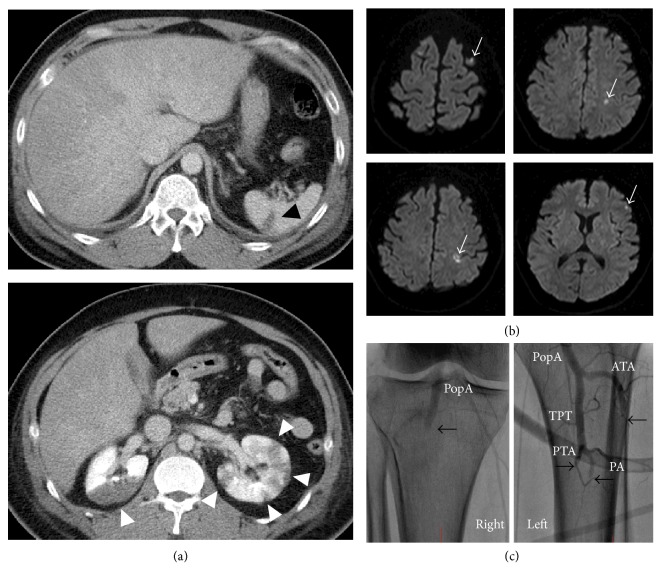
Imaging of systemic embolism. Axial views of contrast enhanced computed tomography on admission showed the splenic (black arrow head) and renal (white arrow head) embolisms (a). Also, head magnetic resonance imaging (diffusion weighted image) revealed cerebral infarctions (white arrow), which suggested they were due to shower cardiogenic embolism (b). Angiograms of bilateral below-the-knee arteries showed occlusions in the right popliteal artery (PopA), left anterior tibial artery (ATA), left peroneal artery (PA), and left posterior tibial artery (PTA) ((c); black arrows). TPT: tibioperoneal trunk.
